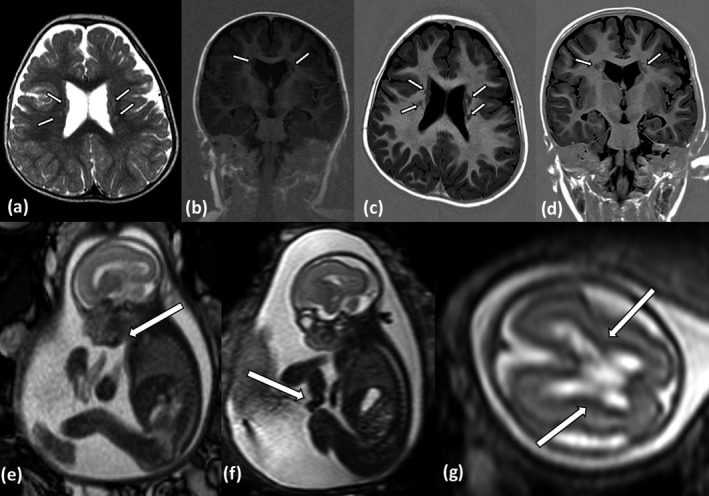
Index patient: (a,b) cranial MRI at the age of 17 months, (a) T2 axial, (b) T1 IR coronal; (c,d) Cranial MRI at the age of 2 9/12 years, (c) T1 IR axial, (d) T1 IR coronal. MRI follow‐up shows bilateral subependymal heterotopia with gray matter nodules, as well as deep subcortical heterotopias. The cortex shows areas of excessive infolding and irregularity on both sides which represent a cortical malformation. Fetus of the prior pregnancy: (e–g) MRI findings at the age 23 + 6 gestational week, T2w images. (e) Retrognathia; (f) flexion contracture of the hand; (g) mild periventricular hyperintensity
